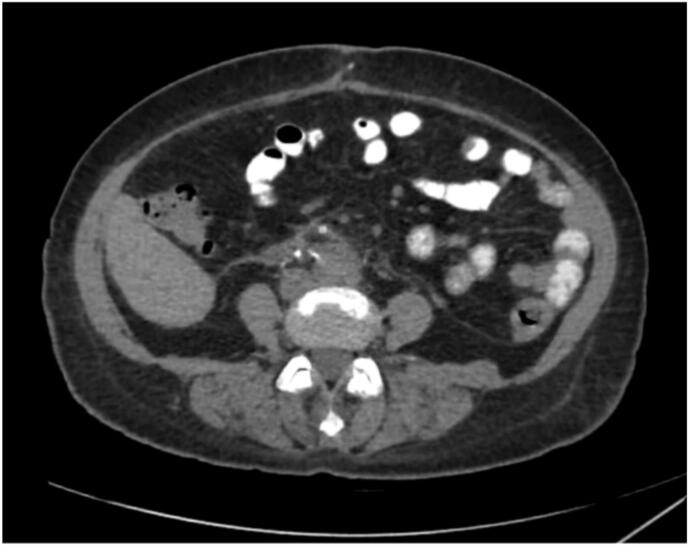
Postoperative CT scan of the abdomen and pelvis, enhanced with PO and IV contrast (axial view), demonstrates the successful removal of the horseshoe kidney and the cystic structures associated with polycystic kidney disease.
